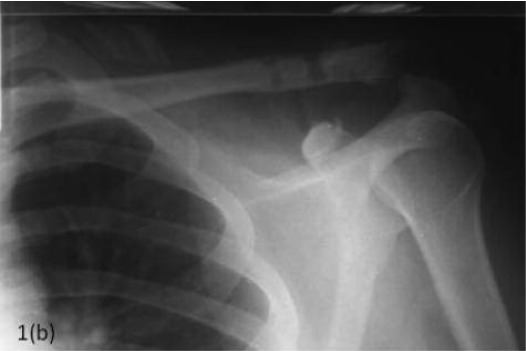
Immediate post operative radiograph of the shoulder
